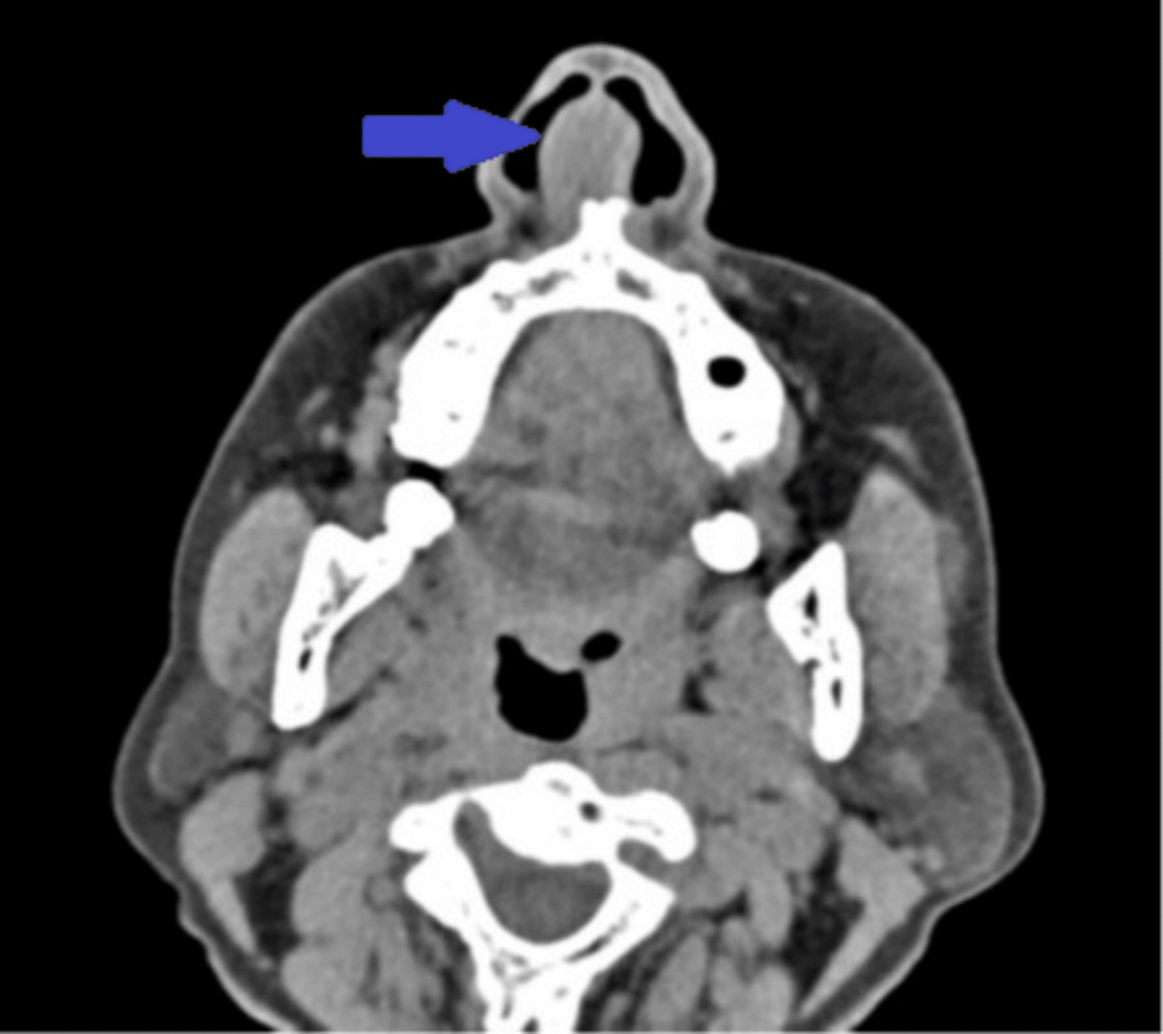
Computed tomography image of the nose and paranasal sinuses showing a solid dense swelling in the septal cartilage
